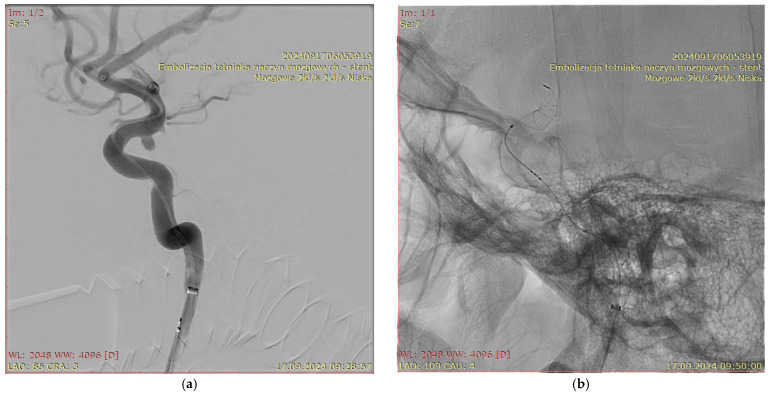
An angiographic image of the intracranial aneurysm found in Patient X (a) and a fluoroscopic X-ray image acquired during stent deployment (b) “mózgowe 2 kl/s, 2 kl/s niska” (“Cerebral 2 fps, 2 fps Low”), “embolizacja tetniaka naczyn mozgowych- stent” (“Endovascular embolization of a cerebral aneurysm- stent”).
